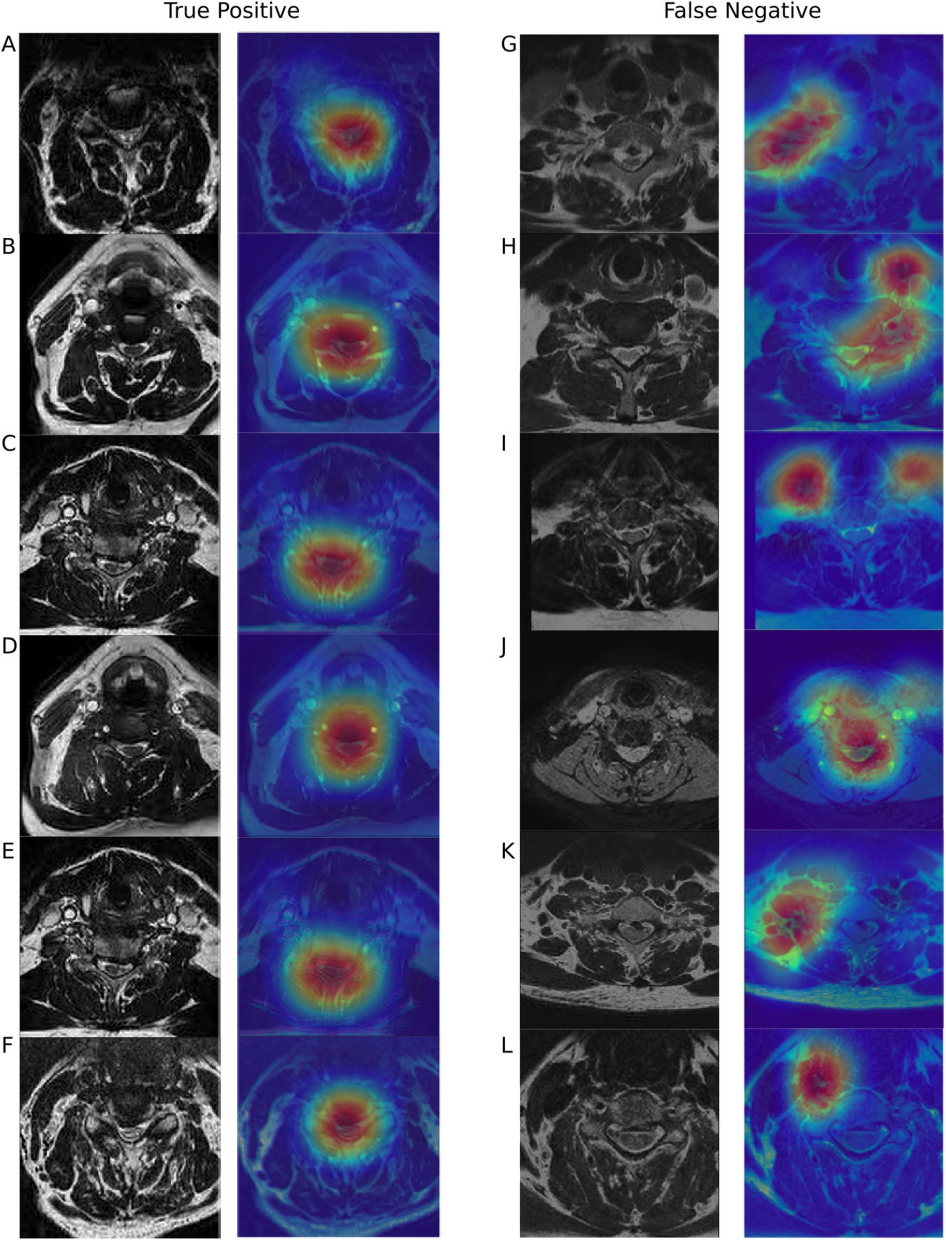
Class activation maps for example images that were classified correctly (true positives) or incorrectly (false negatives). The first column represents the axial T2-weighted MRI image. The second column shows the MRI image with the class activation map overlaid. In the class activation maps blue represents no activation while red represents maximal activation. On these example images of true positive classifications, the maximal activation tended to be over the spinal canal and spinal cord (Images A,B,C,D,E,F). In the false negative classifications, the activation was sometimes over irrelevant areas of the image, such as the paraspinal muscles or vascular structures (Images G,I,K,L). In other examples of false negative classifications there was activation over the spinal cord and spinal canal (Images H and J), but in these cases there was also activation over other seemingly irrelevant areas of the image.
